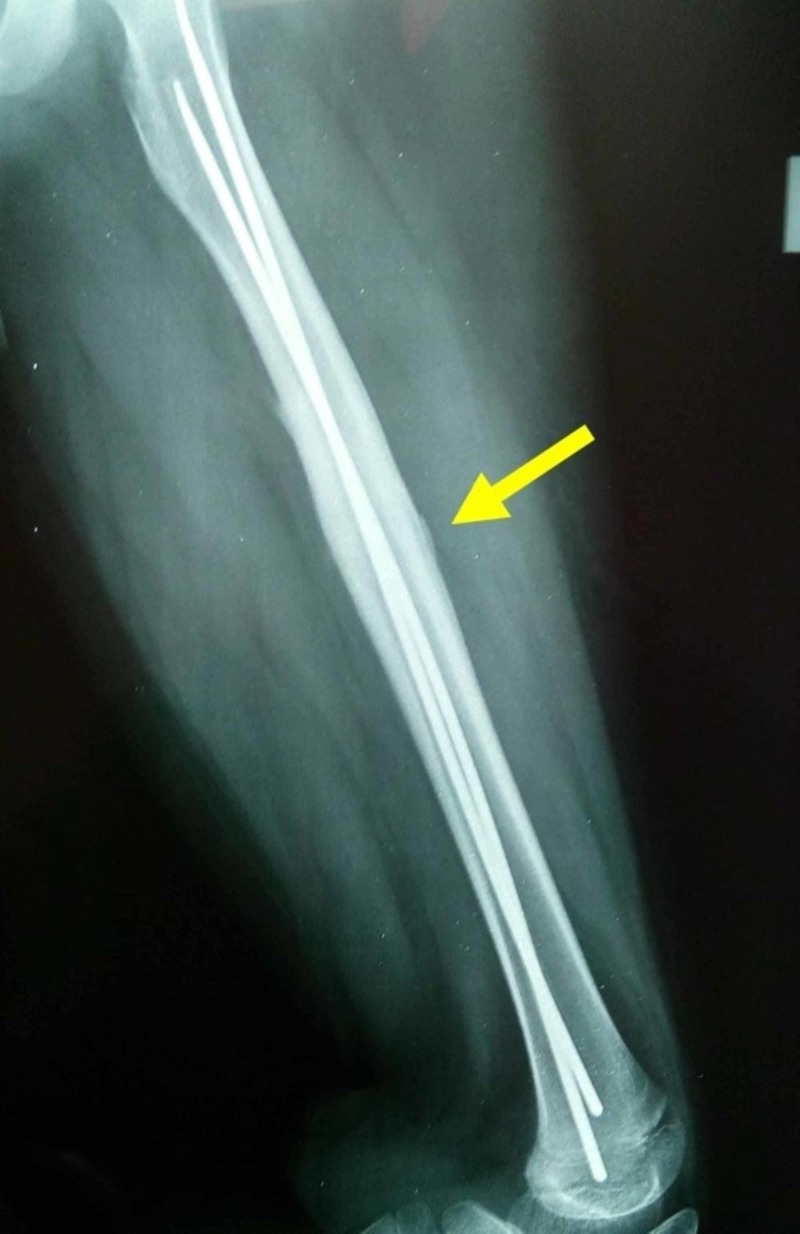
Lateral X-ray view of the operated left femur six months after surgery; fracture healing is complete Arrow indicates complete fracture healing and a partially restored antecurvatum of the left femur.
